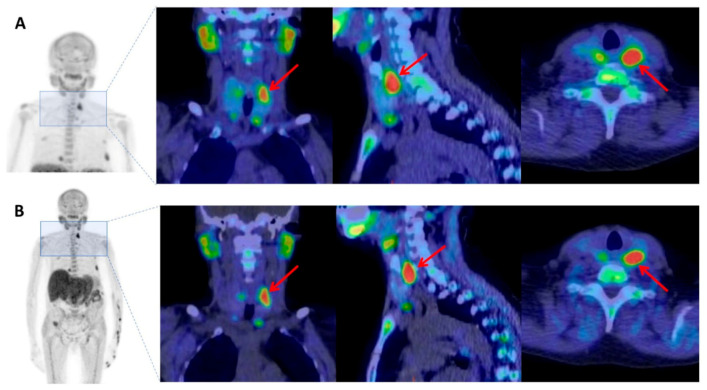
^18^F-FCH PET/CT was performed as the last preoperative imaging. (A) A static image acquisition was started 5 min after injection of 2.5 MBq/Kg of ^18^F-FCH from the skull to the diaphragm in order to include any ectopic foci, based on the literature evidence [5,6]. (B) A late acquisition at 60 min was subsequently performed, choosing a whole-body protocol because of the evidence of ^18^F-FCH uptake in bones in the early scan. PET/CT clearly showed ^18^F-FCH uptake in the four parathyroid glands, particularly in the left superior one that appeared also enlarged (red arrows).
